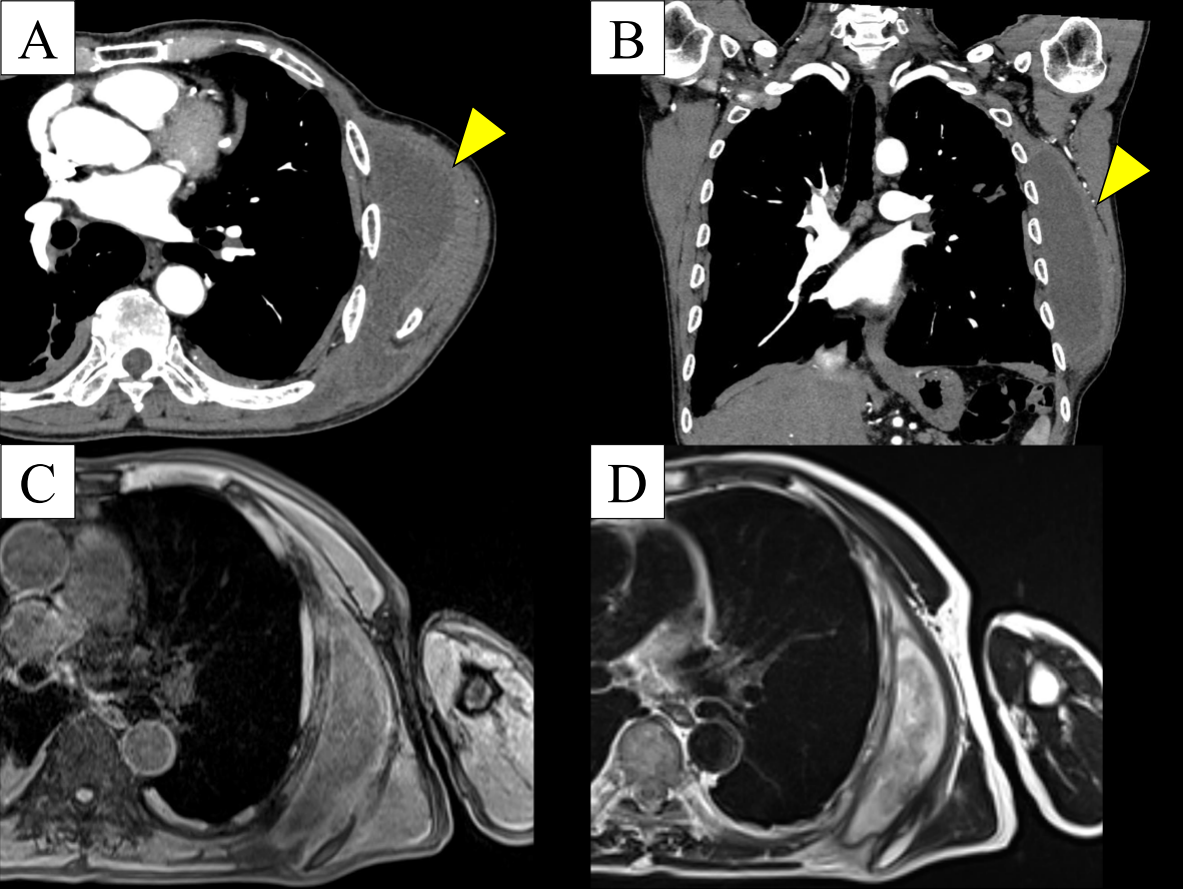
Enhanced computed tomography scan showed a capsulized cystic lesion and substantial ingredients within the lesion (A, B). Contrast-enhanced magnetic resonance imaging revealed a lesion with a viscous liquid (C T1W2, D T2W1)
